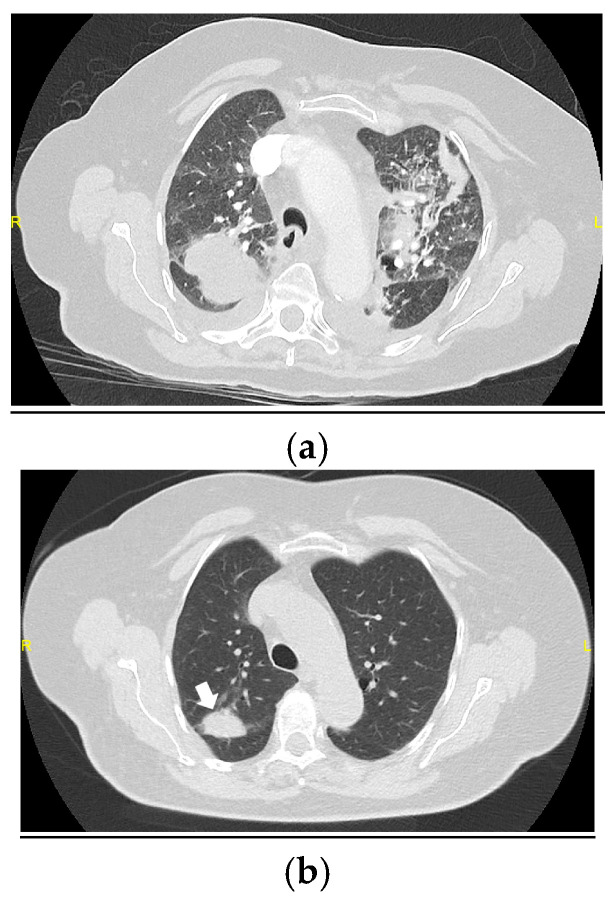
(a) HRCT performed 6 months after hospital discharge, showing diffuse ground-glass opacities, multilobar parenchymal consolidations with a predominantly central distribution and bilateral pleural effusion. (b) HRCT at 12 months, demonstrating the near-complete resolution of pulmonary abnormalities, except for a small persistent consolidation in the right upper lobe (arrow).
